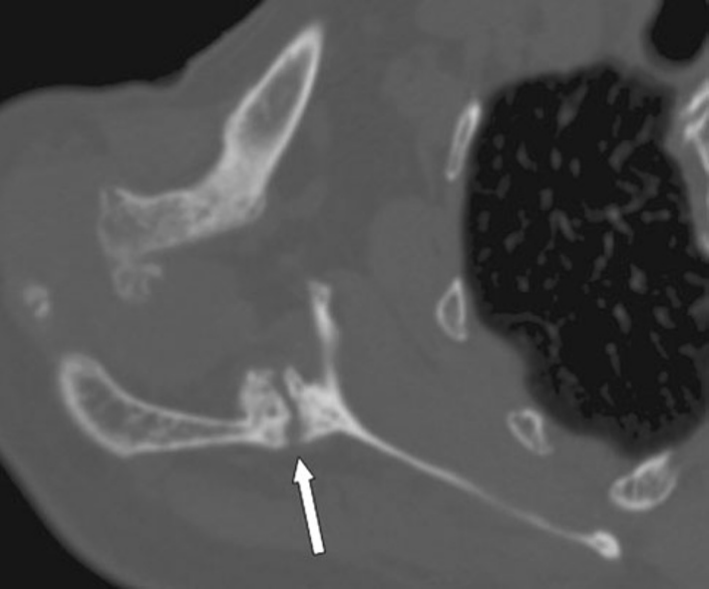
(Patient 2) CT scan of scapular spine fracture, showing callus formation but no consolidation
